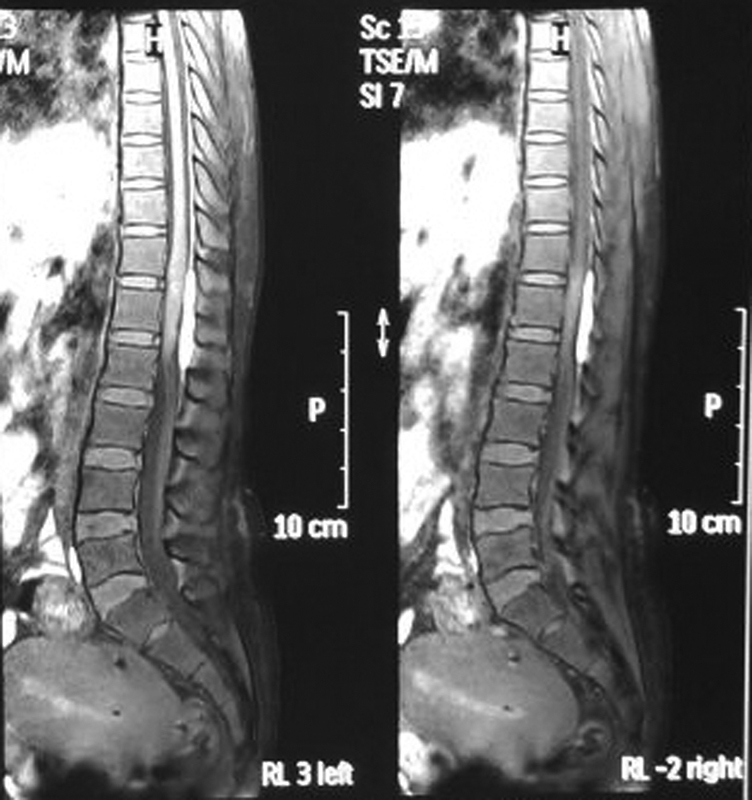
MRI spine sagittal cuts showing extradural broad based ellipsoid lesion measuring 50 × 9 mm along T12–L2 vertebra displacing the dura anteriorly and T1 hyperintensity. L, lumbar; MRI, magnetic resonance imaging; RL, right to left; sc, section; sl, sliceo; T, thoracic; tse, turbo spin echo.
